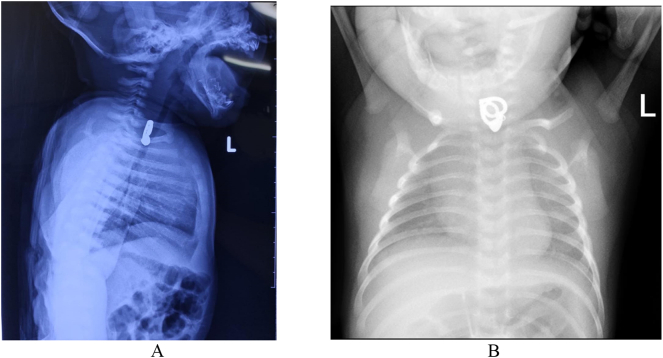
A and B. Chest X-ray show the foreign body at the level of the 7th Cervical vertebrae - 1st Thoracal behind the trachea.
